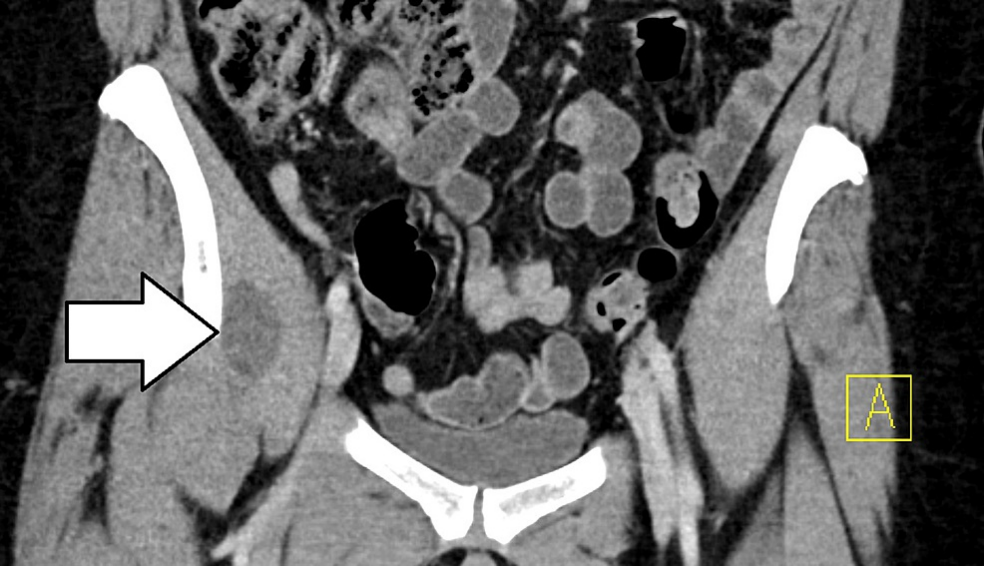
CT with contrast (coronal image) with right-sided iliopsoas bursitis. CT: computed tomography.
